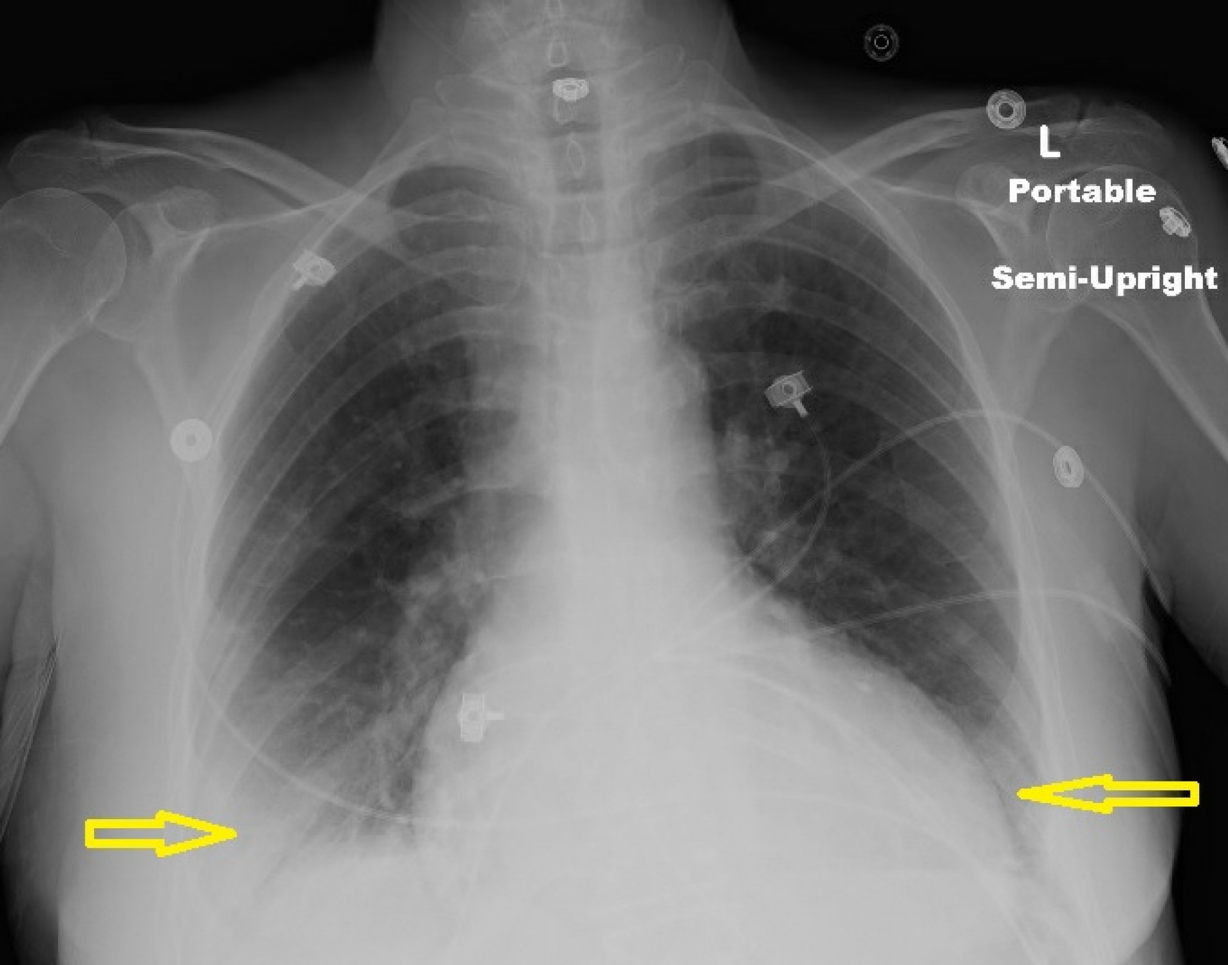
Chest x-ray showed bilateral pleural effusion greater on the right side with evidence of pulmonary vascular congestion.
